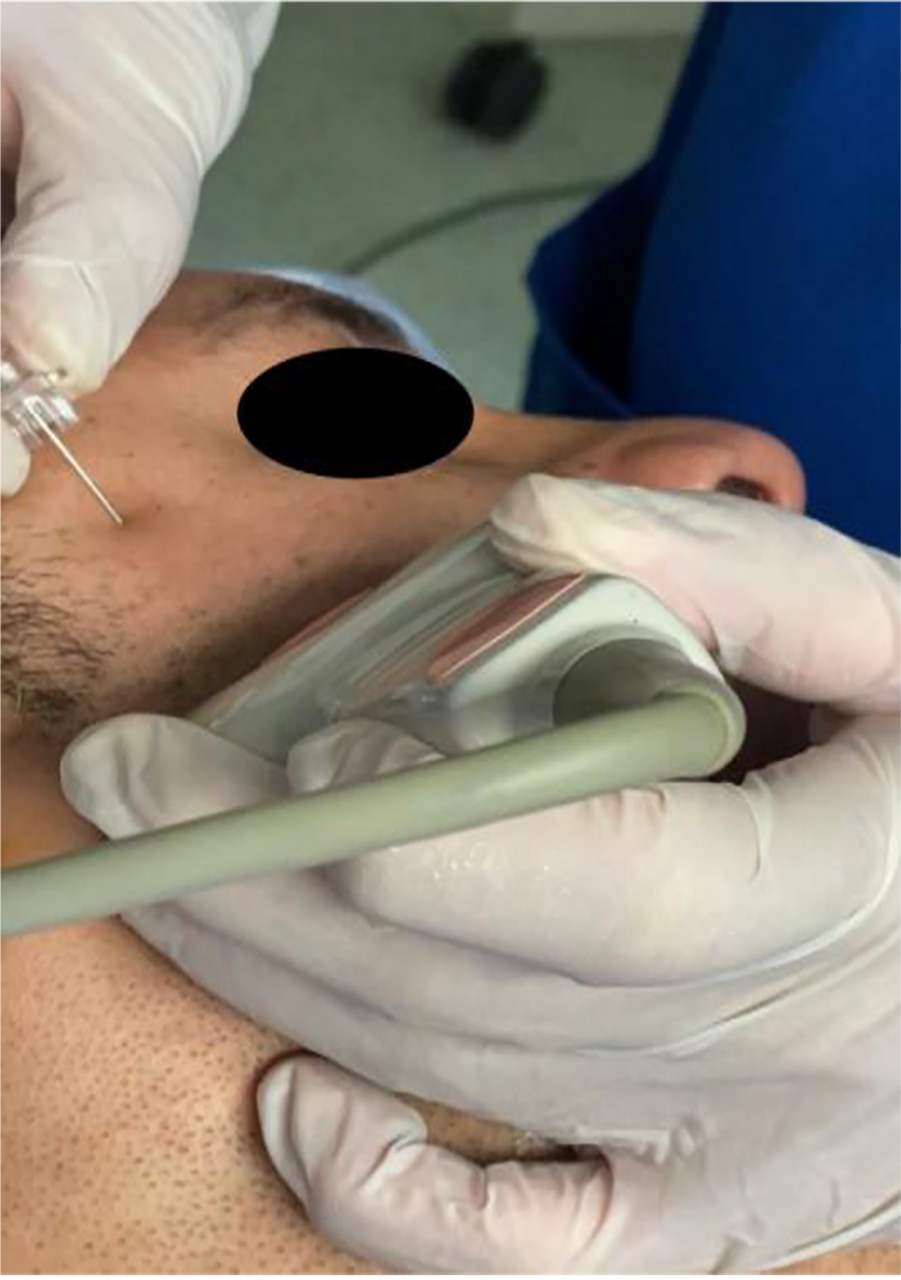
The ultrasound probe in the infrazygomatic area, with an inclination of 45 and the needle at the frontozygomatic angle and redirected to the PPF
